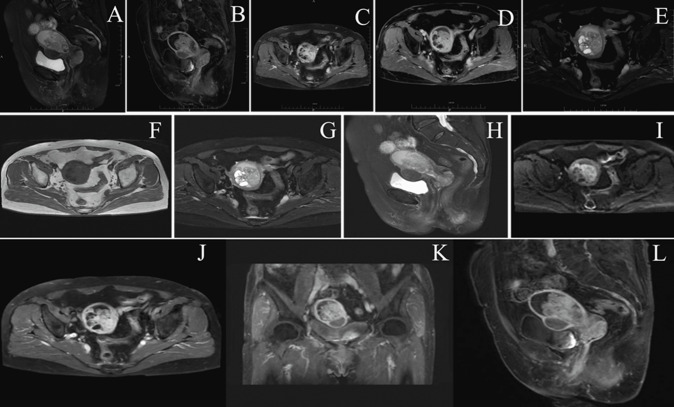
pelvic MRI of adenosarcoma: AB) T1 coronal/sagittal; CD) T2 coronal/sagittal; E) WI; FG) T1 and T2 axial show mixed signal filling uterus; H) T2 sagittal-84 mm cervical mass, uterine involvement, fluid; I) DWI-solid restricted, cystic free; JL) post-contrast solid avid enhancement
